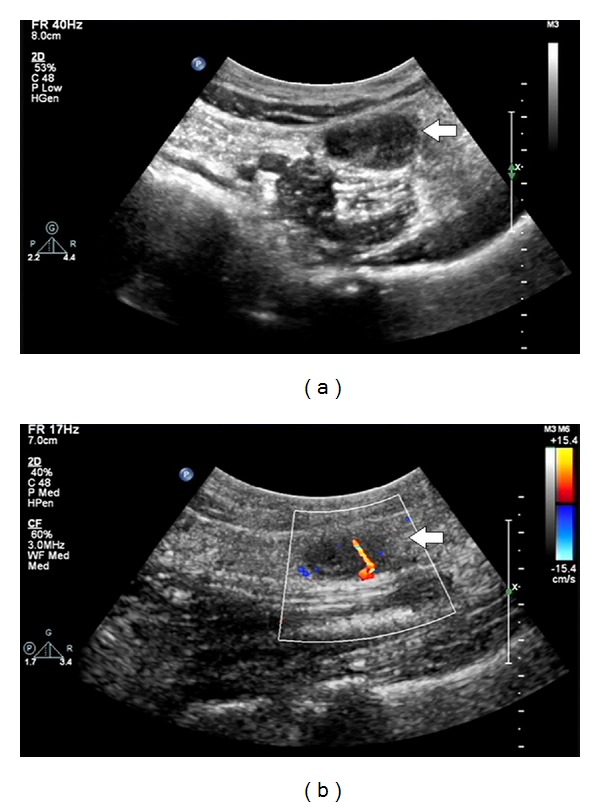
Grey-scale ultrasonography shows (a) a mild heterogenous hypoechoic mass with posterior acoustic enhancement anterior to the left psoas muscle with increased flow within the mass on color Doppler imaging (b) (arrow).
